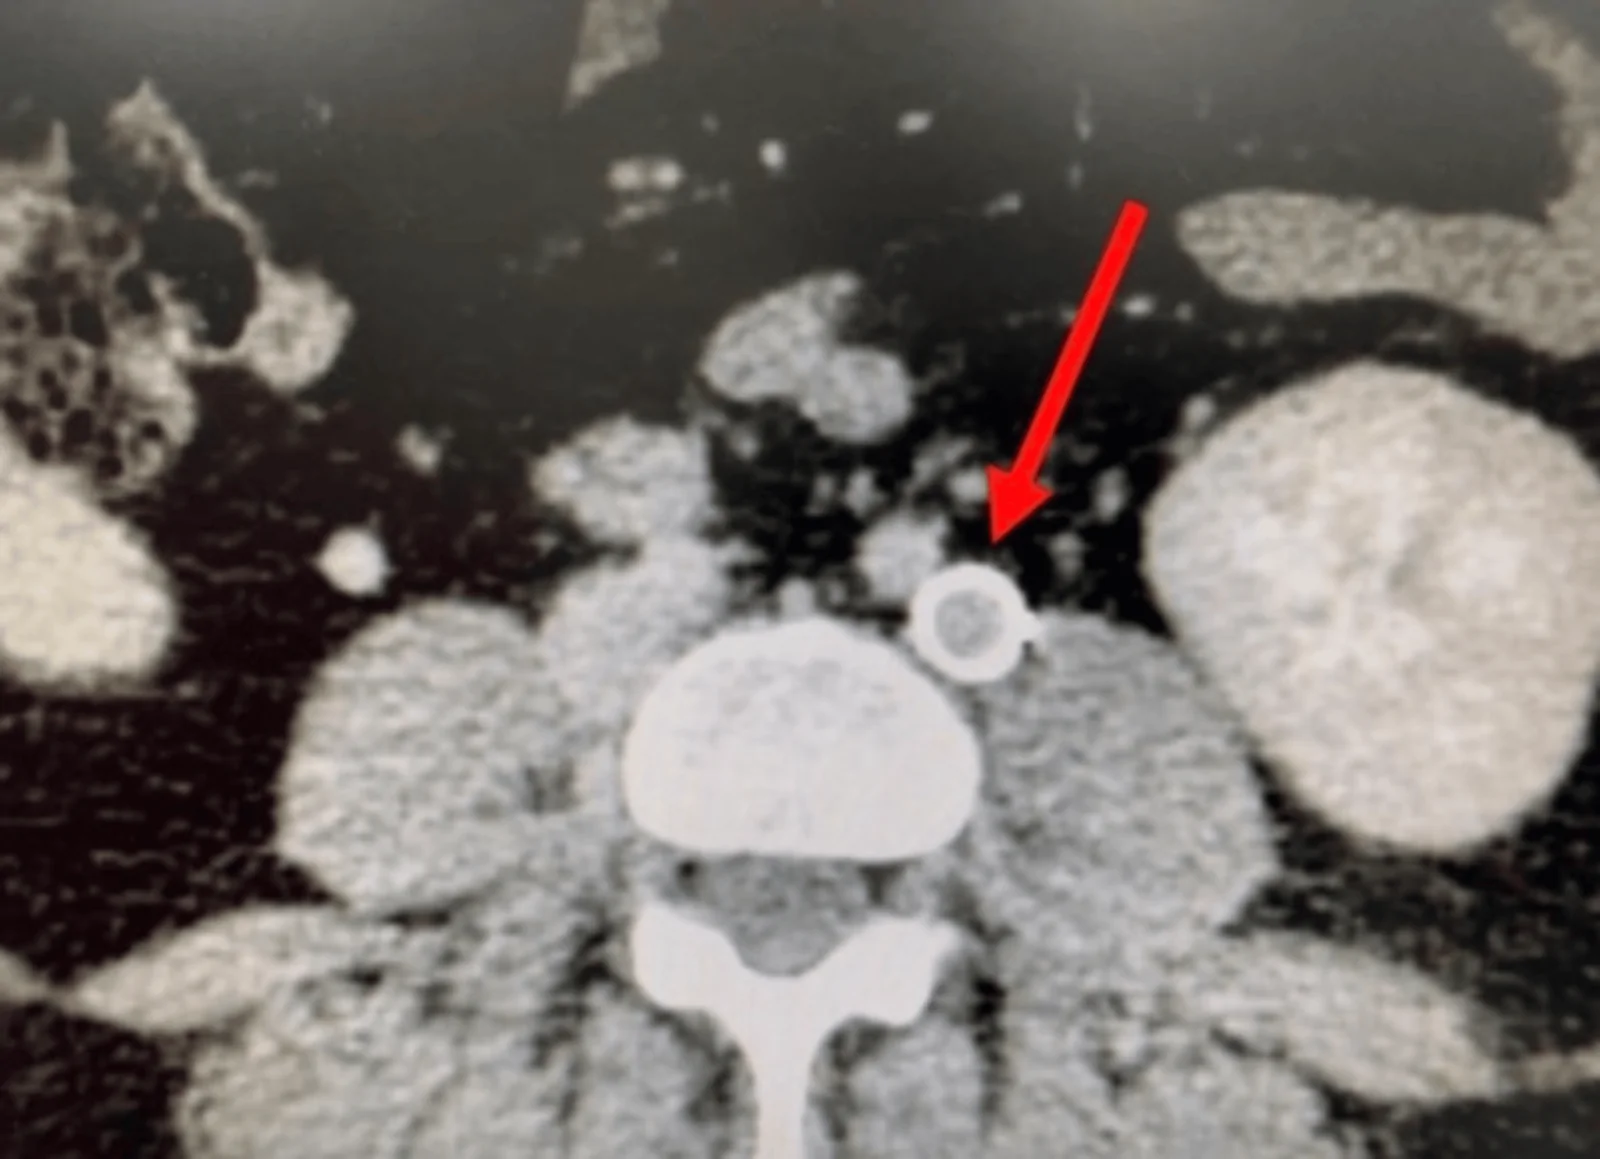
Computed tomography angiography demonstrating stent placement in a duplicated IVC. Axial computed tomography angiography of the abdomen demonstrates a patent endovascular stent within the duplicated inferior vena cava. The red arrow indicates the stented left-sided inferior vena cava, with preserved luminal caliber and contrast opacification confirming vessel patency following the intervention. IVC: Inferior vena cava.
